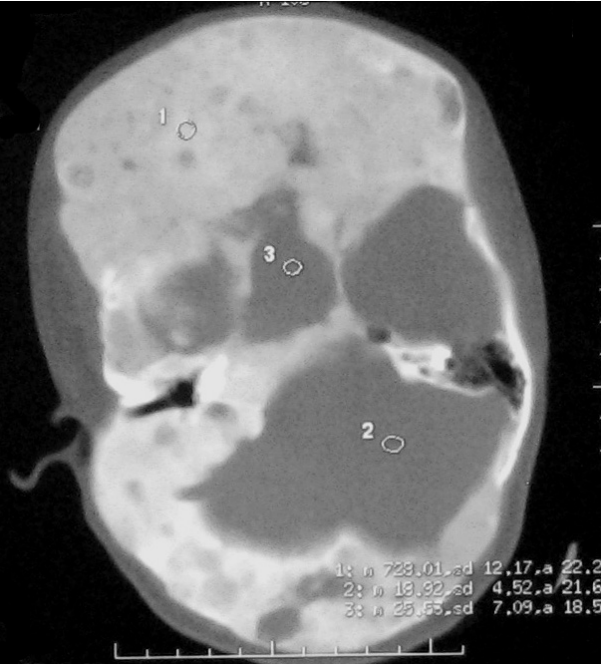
Axial CT image FD presents as "ground-glass" appearance on CT and there is a cystic and solid mass of sella turcica.
